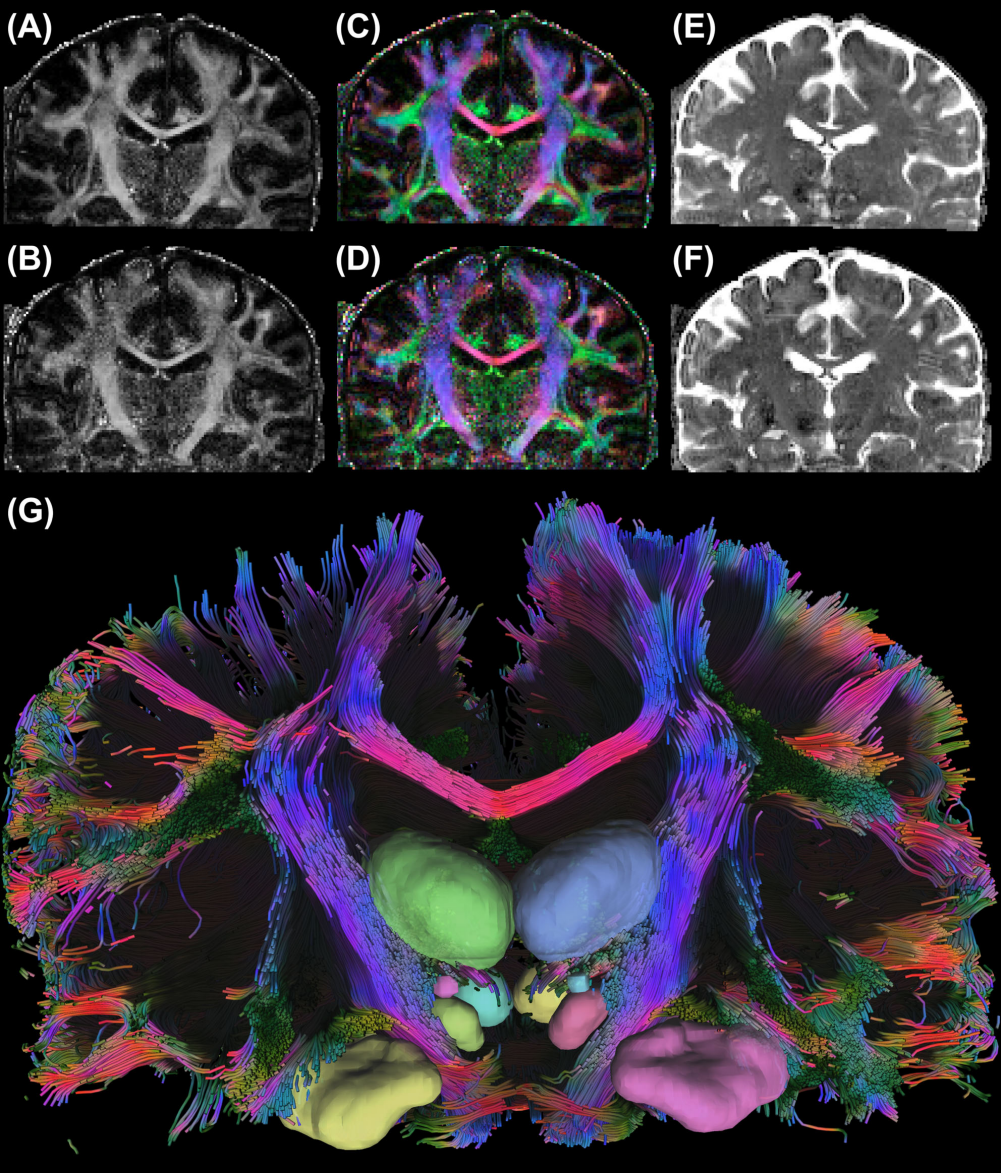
Diffusion MRI metrics following distortion correction using TOPUP and EDDY from FSL. (A–F) Top and bottom rows correspond to data acquired with the 16Tx/80Rx and 16Tx/128Rx coils, respectively. (A, B) Fractional anisotropy maps, (C, D) Primary diffusion orientation maps, and (E, F) Mean diffusivity maps. (G) Tractography from the 16Tx/128Rx dMRI dataset, depicting major association, projection and commissural tracts, including the superior longitudinal fasciculus, corticospinal tract and corpus callosum.
